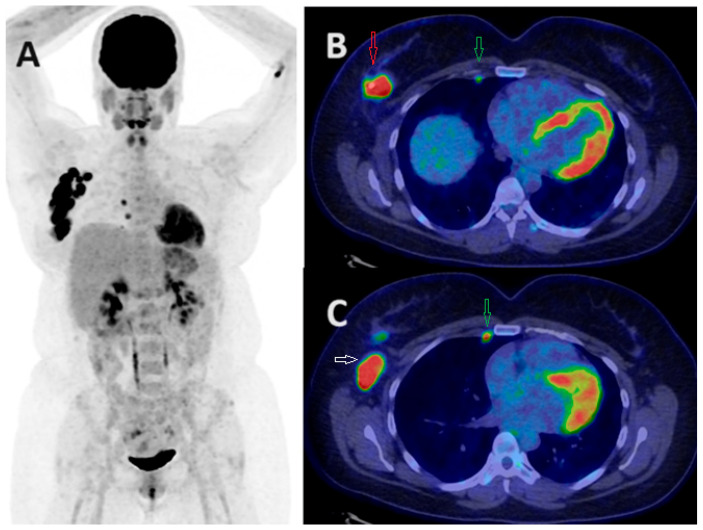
FDG PET/CT in BC nodal staging. The PET/CT MIP (A) and axial fused PET/CT (B,C) images demonstrate a hypermetabolic right breast primary (red arrow) with multiple hypermetabolic ipsilateral right level I/II axillary (white arrow) and internal mammary (green arrow) nodal metastases.
